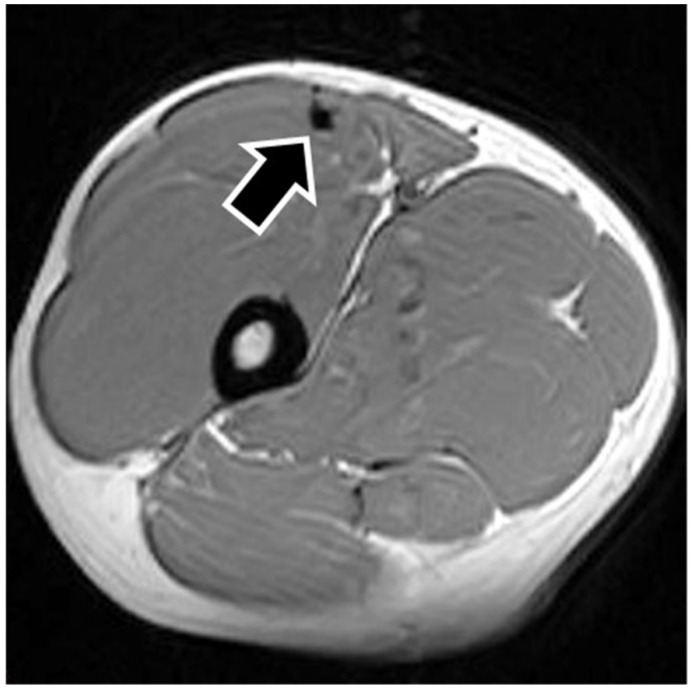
Pseudocyst of the central tendon. The figure shows an example of central tendon injury. The axial T1-weighted image shows a low signal surrounding the central tendon (black arrow), representing fibrous encasement.
